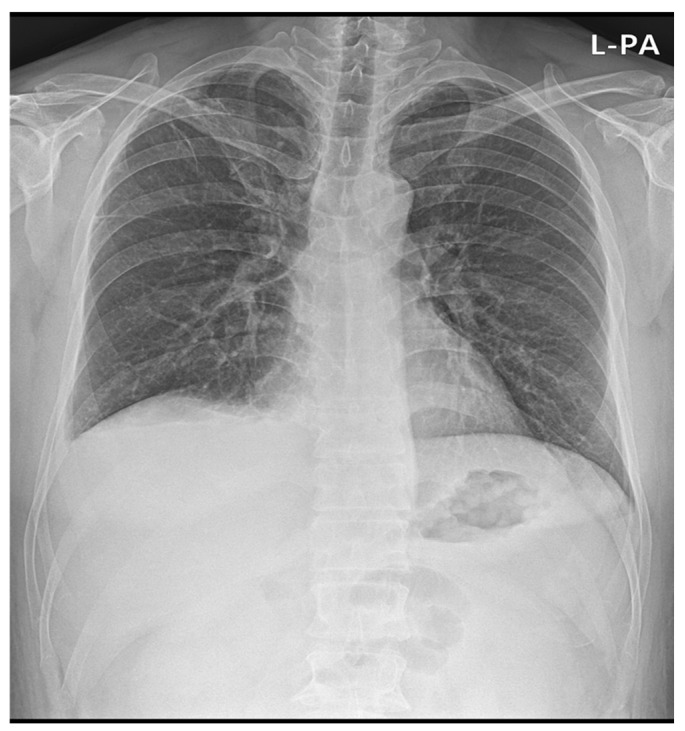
Chest X-ray of the patient showing pleural effusion.
